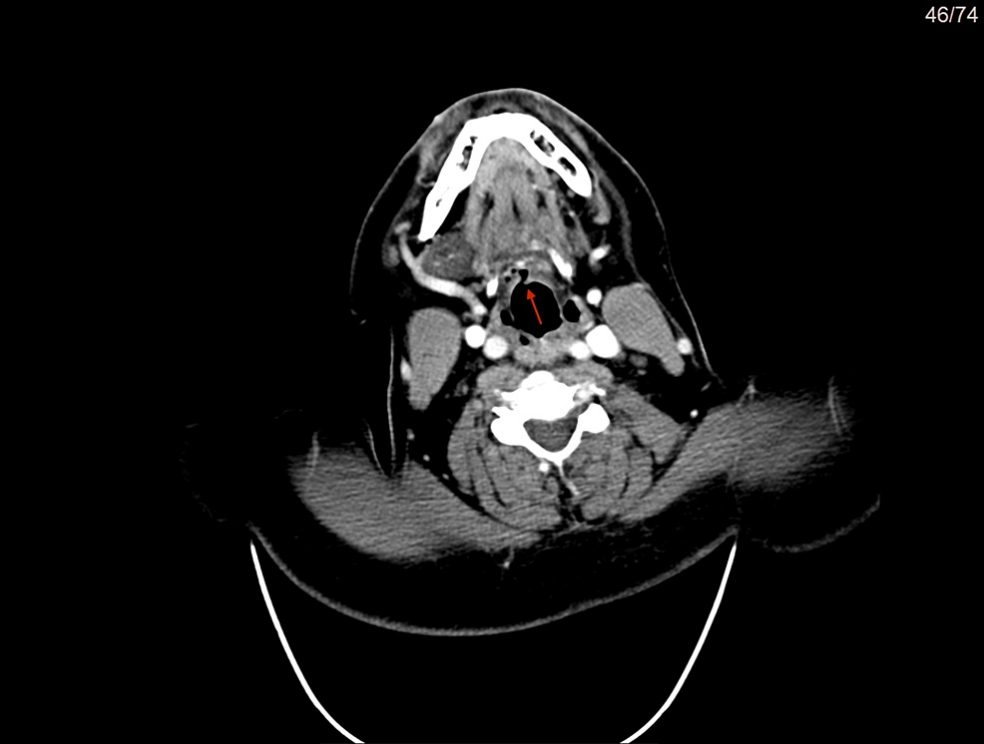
CT scan in axial view of the bifid epiglottis (red arrow)
